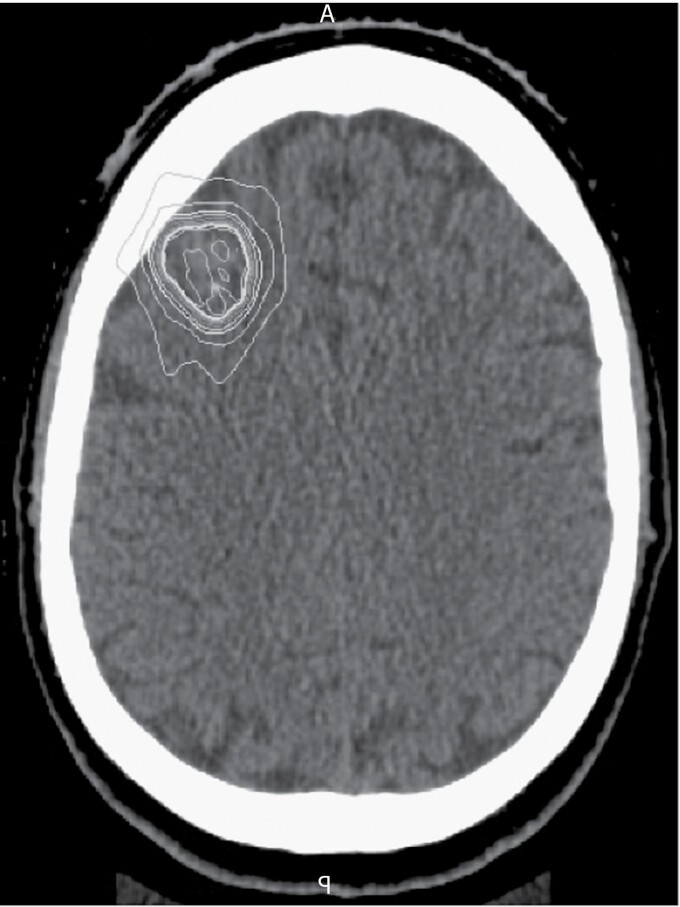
Representative radiation isodose lines for planning post-LITT stereotactic radiotherapy, corresponding to the first case in Figure 1. Obtained from CT scan, the most central line denotes 1890 cGy radiation and the most superficial 540 cGy. LITT, laser interstitial thermal therapy.
